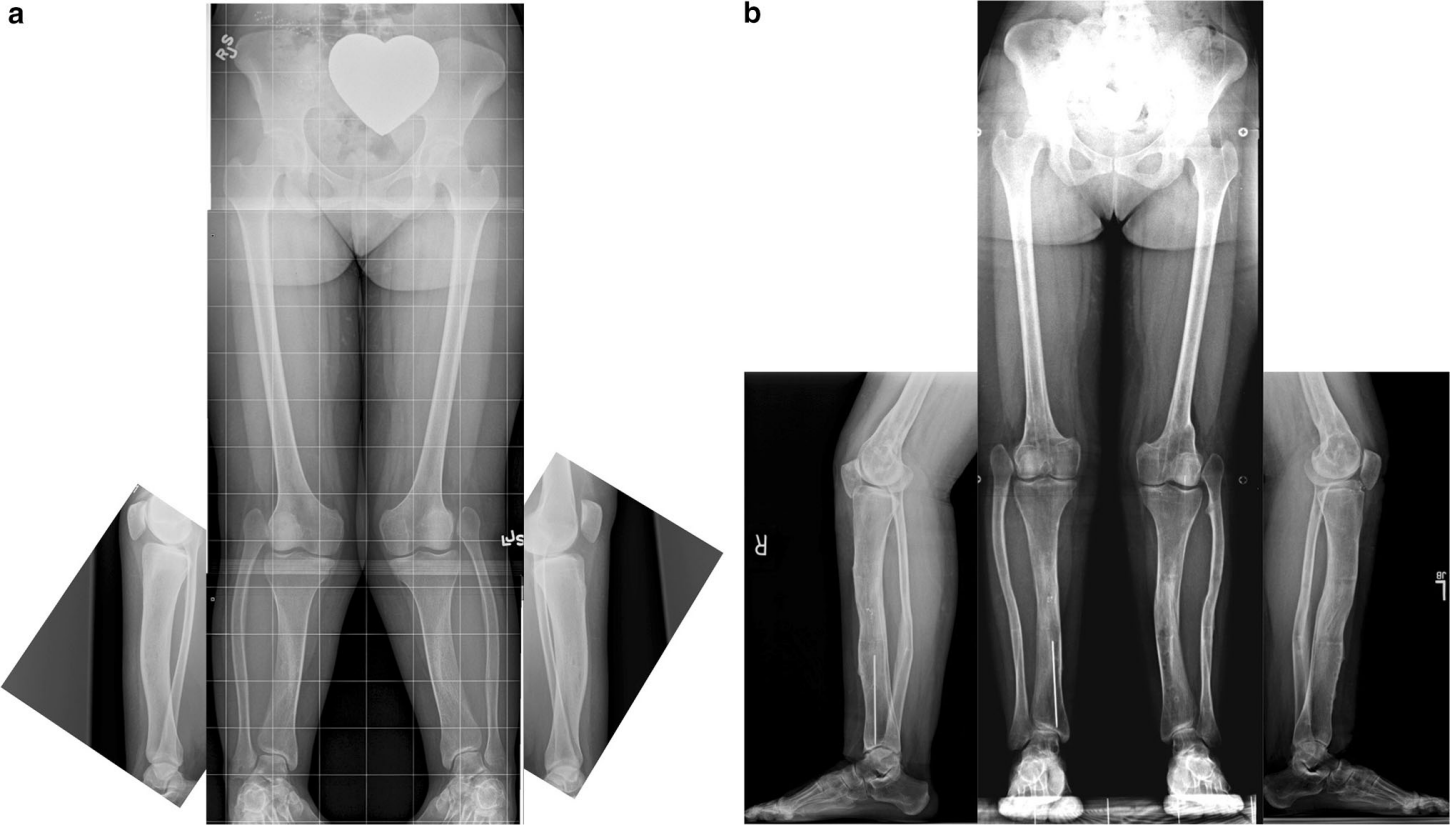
a Antero-posterior (AP) and lateral radiographs of 20-year-old woman with bilateral Paley type 1 tibial hemimelia. The tibia is well formed at both the knee and ankle joints. The fibulas are relatively overgrown at their proximal ends and are articulating with the side of the femurs. The knees are both in valgus due to both tibial and femoral deformities. The tibias have mild procurvatum diaphyseal bowing. Since both tibias are relatively short compared to the femurs, the patient has a mesomelic disproportion and short stature. b AP and lateral radiographs after treatment. Both tibias were lengthened with external fixators. The tibial valgus-procurvatum was corrected. The fibulas were not pulled down. Bilateral femur varus osteotomies were performed after completion of the tibial correction. The lengthening restored the proportion of the tibias and femurs to normal
